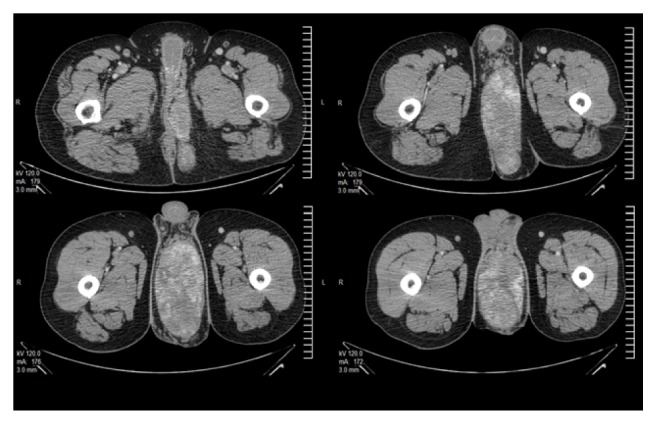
CT scan showing a large smoothly marginated perineal mass with uniform soft-tissue attenuation.
